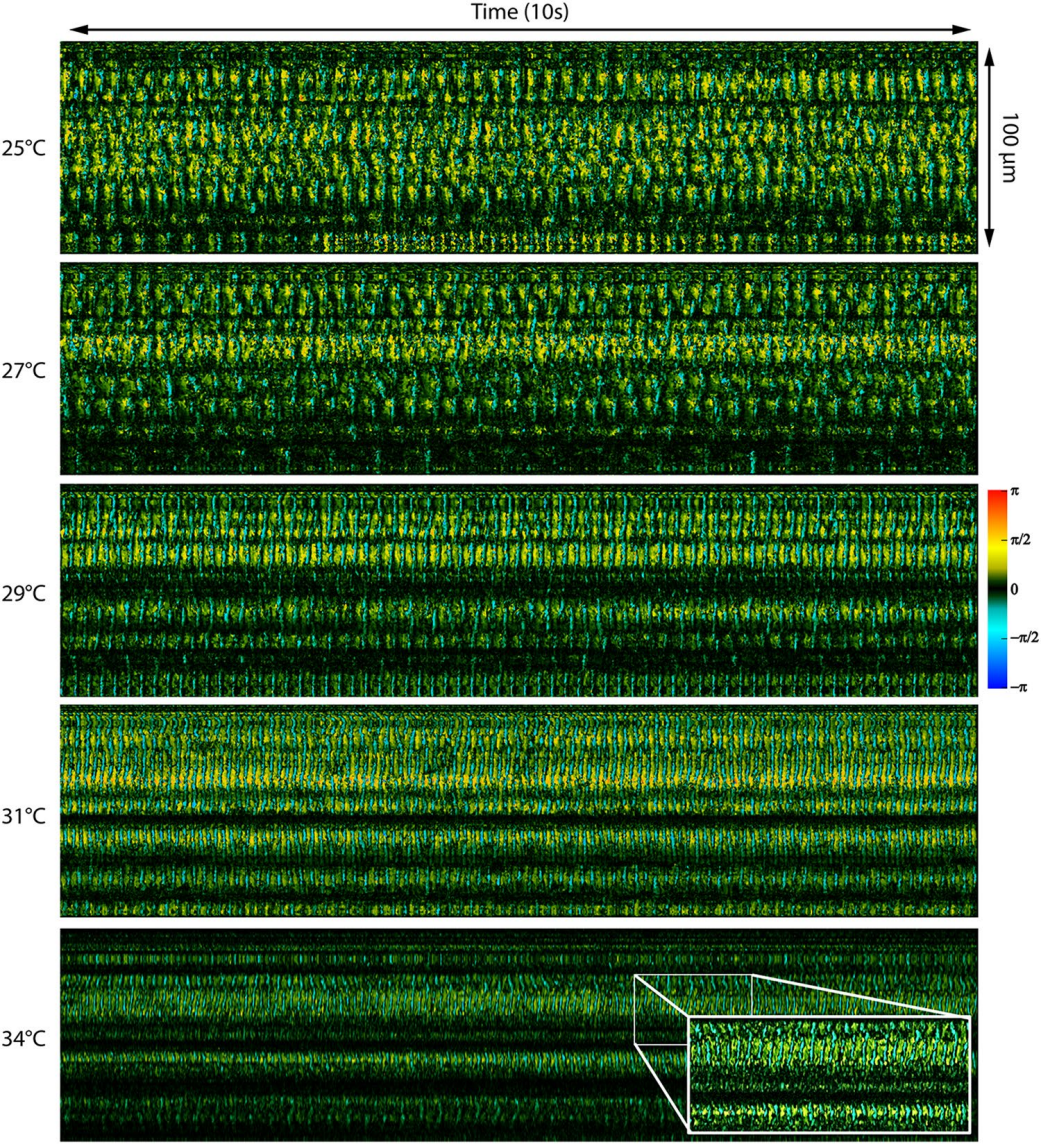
Doppler images of ciliary motion over time. Acquired at temperatures of 25 °C, 27 °C, 29 °C, 31 °C, and 34 °C. 10 seconds duration. Red/yellow represents periods of upward motion while blue/turquoise represents periods of downward motion.
